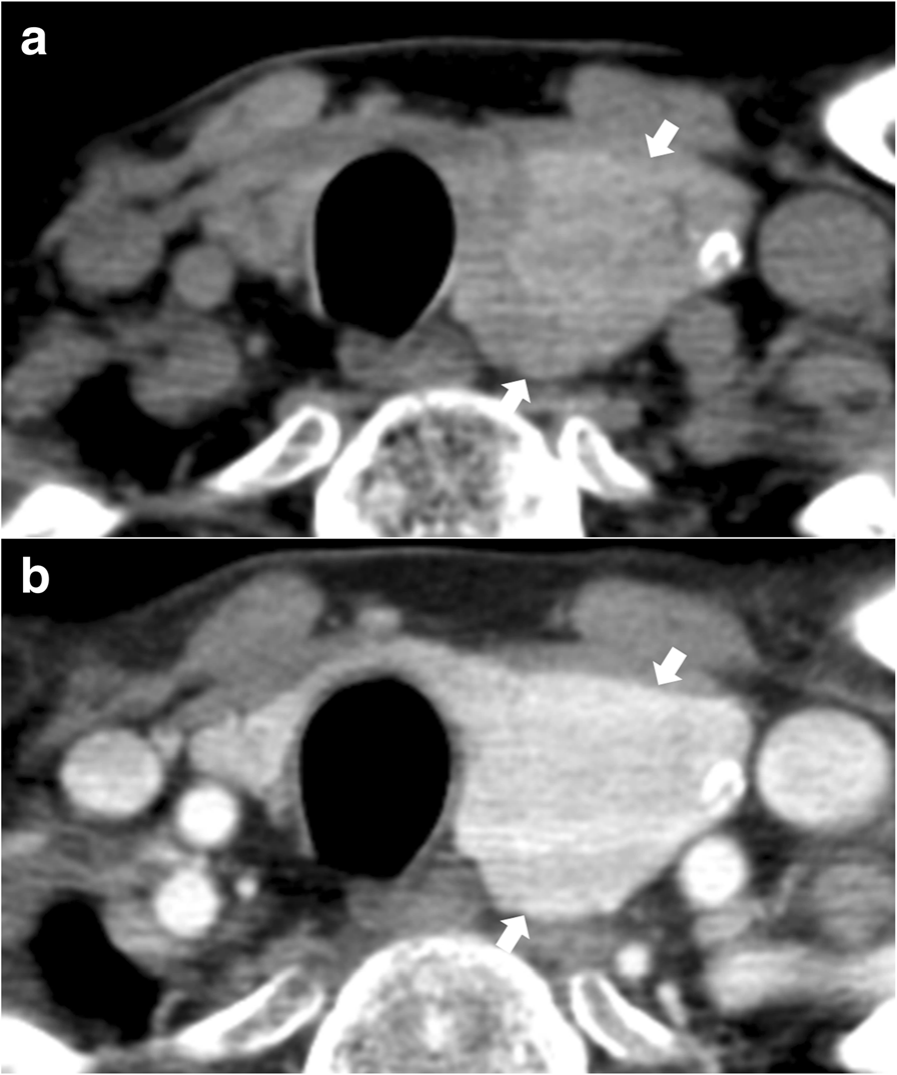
A 62-year-old man with nodular hyperplasia in the left thyroid lobe (largest diameter, 3.5 cm). In non-enhanced (a) and contrast-enhanced (b) axial CT images, nodular hyperplasia (arrows) in the left thyroid lobe shows inhomogeneous iso-attenuation, ovoid shape, homogeneous iso-enhancement, and absence of the CT halo sign
